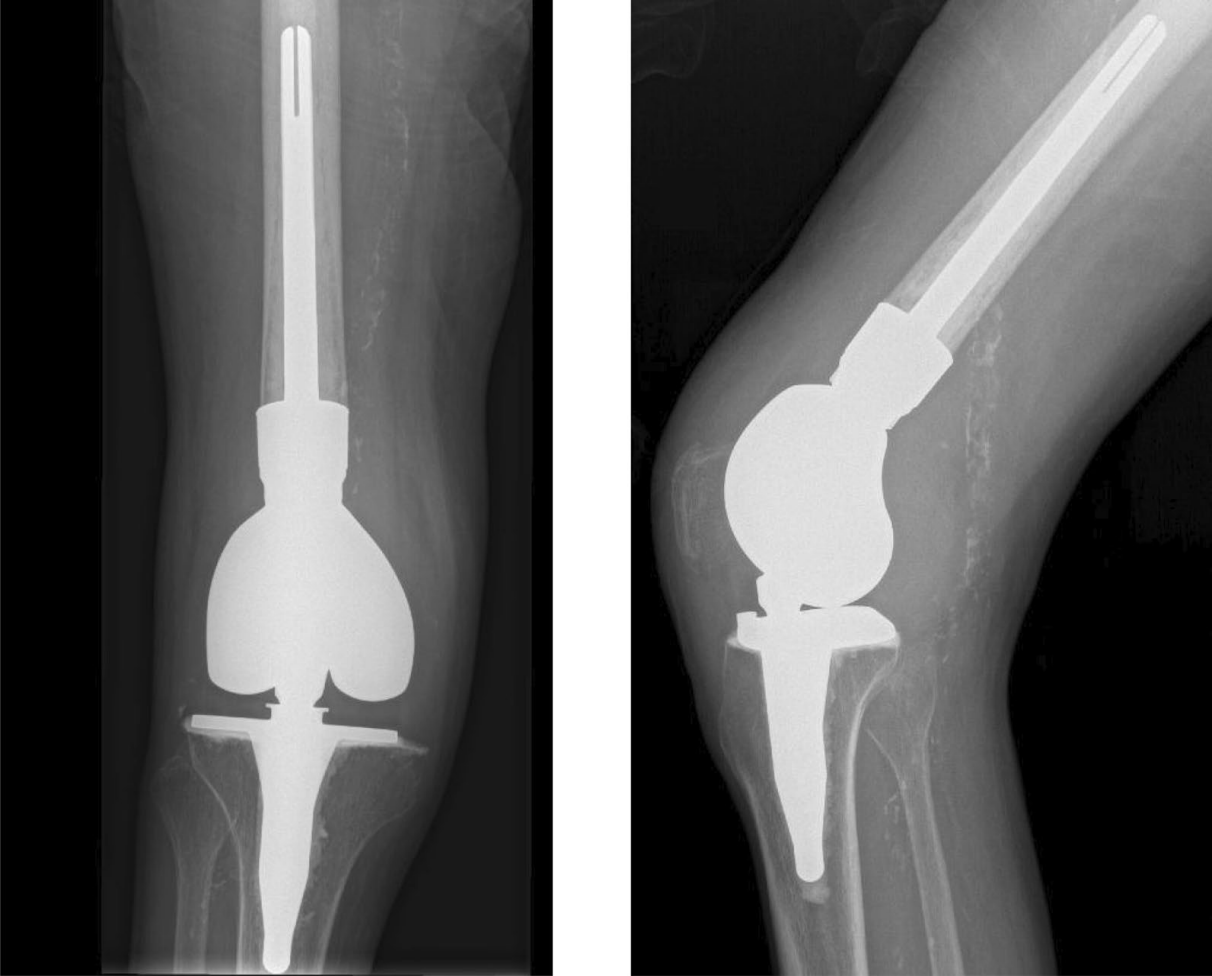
AP (left) and lateral (right) radiographic imaging of a distal femoral replacement megaprosthesis
